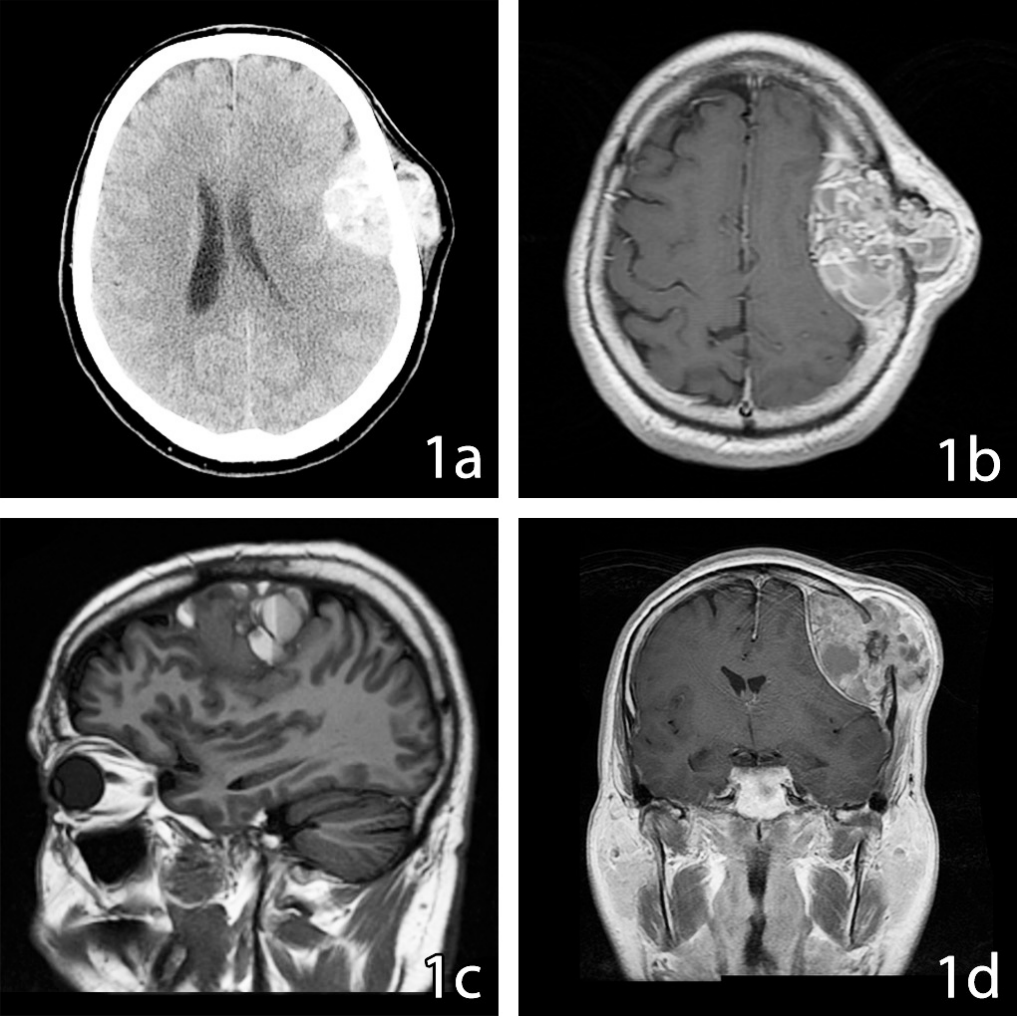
Skull Tumor Aggressive osteoblastoma imaging before surgical resection. 1a: Axial CT. 1b: Axial T1-weighted MR. 1c: Parasagital T1-weighted MR. 1d: Coronal T1-weighted MR. High attenuation mass with intracranial and extracranial components on CT (1a) appears well circumscribed. The lesion has heterogeneous intensity on T1-weighted MR (1b), predominantly hyperintense with isointense areas, compromises the integrity of left parietal bone (1c) and produces a slight midline shift to the right (1d). CT: computed tomography MR: magnetic resonance
